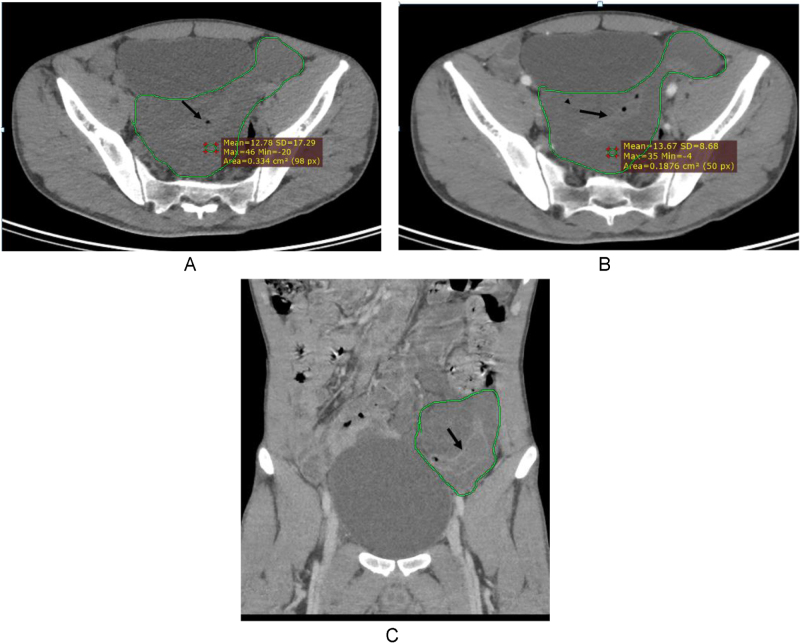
Contrast-enhanced abdominal CT scan showing a large, well-defined hypodense multiloculated cystic lesion in the small bowel mesentery. (A) Pre contrast axial CT, shows a hypodense mass in the retrovesical and paravesical region through which the bowel traverses (black arrow – traversing bowel, green ring – the mass). (B) Post-contrast axial scan, the mass has no post-contrast enhancement, but the traversing bowel has wall enhancement (black arrow – traversing bowel, green ring – the mass). (C) Post-contrast coronal image, the mass has no enhancement on post-contrast study, but the traversing bowel has wall enhancement (black arrow – traversing bowel, green ring – the mass).
